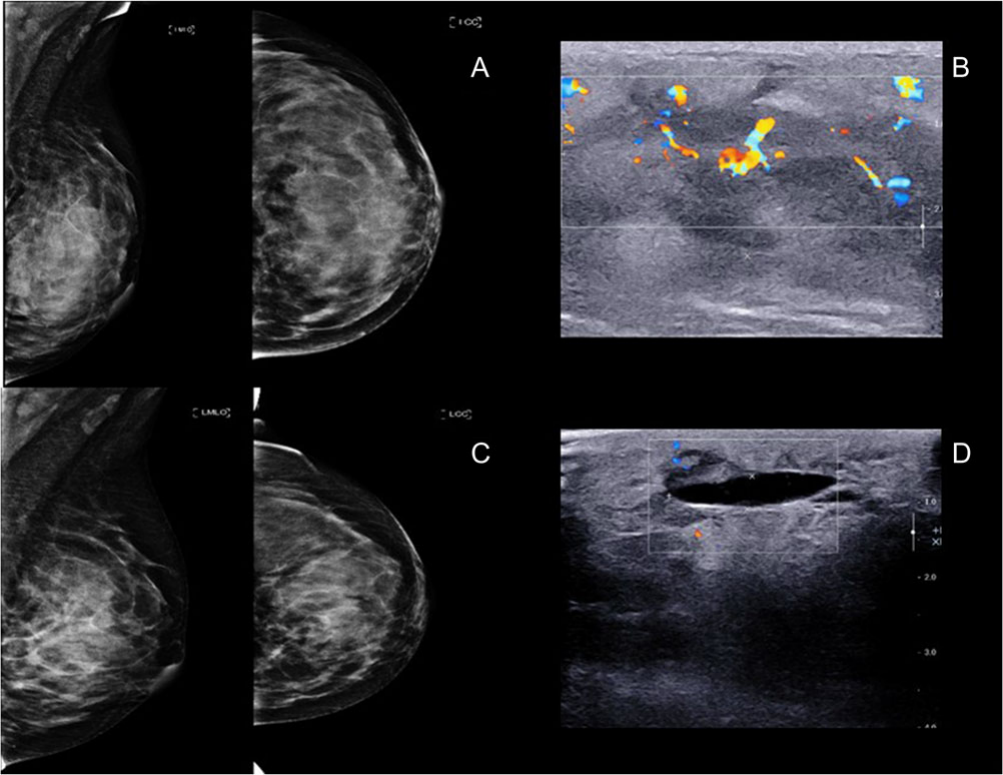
(A and B) Mammogram and ultrasound showed an ill-defined big mass at left mid inner quadrant with hypervascularity, subdermal edema and overlying skin thickening. (C and D) Mammogram and ultrasound post radiation showed 5.4 × 2.4 cm^2^. Ill-defined mass at left upper to mid inner quadrant with interval decreased internal hypervascularity.
